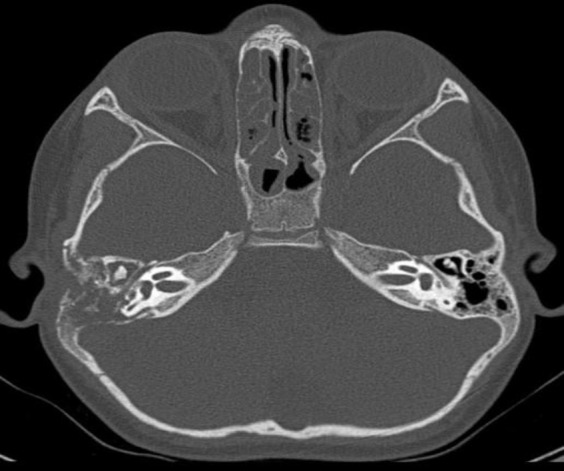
Pre-operative HRCT Temporal Bone showing a soft tissue density in the right external auditory canal and middle ear with erosion of bone
